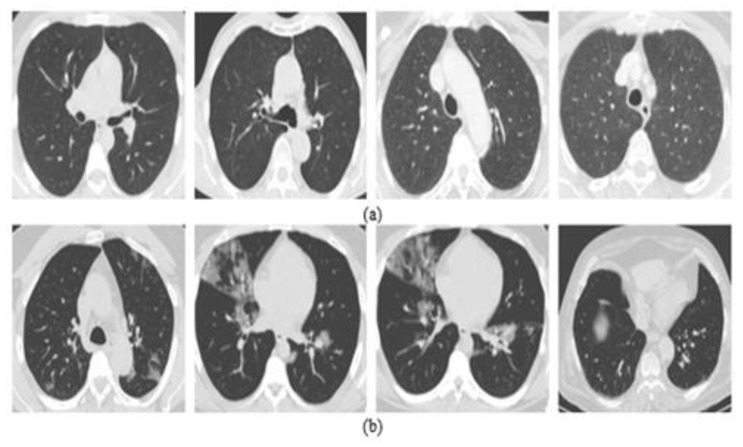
Samples of patients from the CT dataset: (a) non-COVID-19 and (b) COVID-19.
